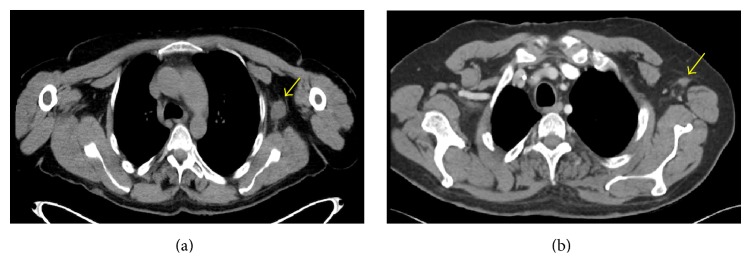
Treatment effect of an axillary MPNST following neoadjuvant chemotherapy. CT chest of Patient 1 revealing the left axillary MPNST (arrow) prior to treatment (a) and after five cycles of neoadjuvant chemotherapy (b). Note that patient's arms were not in the same position in each scan causing the tumor to be in a slightly different location.
